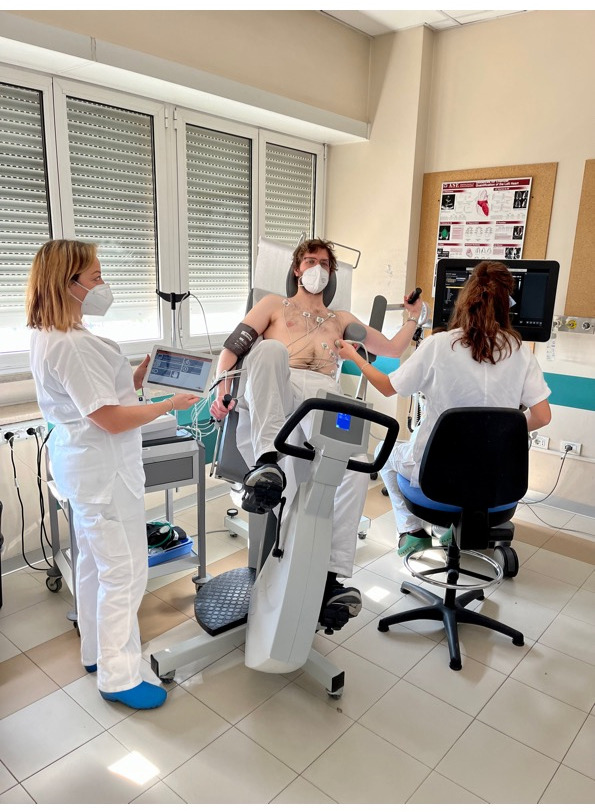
Echocardiographic data acquisition with semi supine bicycle.
